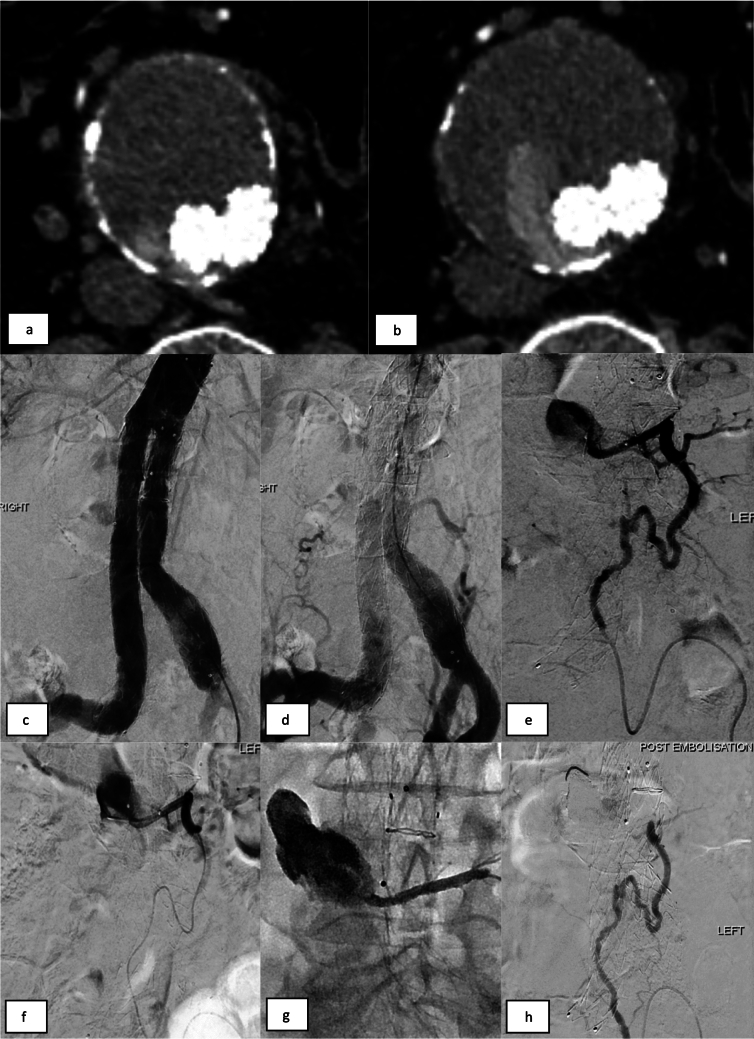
Transarterial embolisation of a left lumbar artery T2EL. (a, b) CTA imaging demonstrates a T2EL arising from a left lumbar branch with contrast filling the aneurysm sac posteriorly and around the right iliac limb endograft. (c-e) Catheter angiograms from the aorta and left iliolumbar artery demonstrate retrograde filling of the aneurysm sac via the left lumbar branch. (f) A 2.7Fr microcatheter was advanced into the aneurysm sac and an angiogram confirms catheter tip position and excludes communication with any spinal branches. (g) 9 ml of EVOH (Onyx 34L) injected via the microcatheter spreads into the sac and inflow lumbar vessel. (h) Completion angiogram confirms no further endoleak
